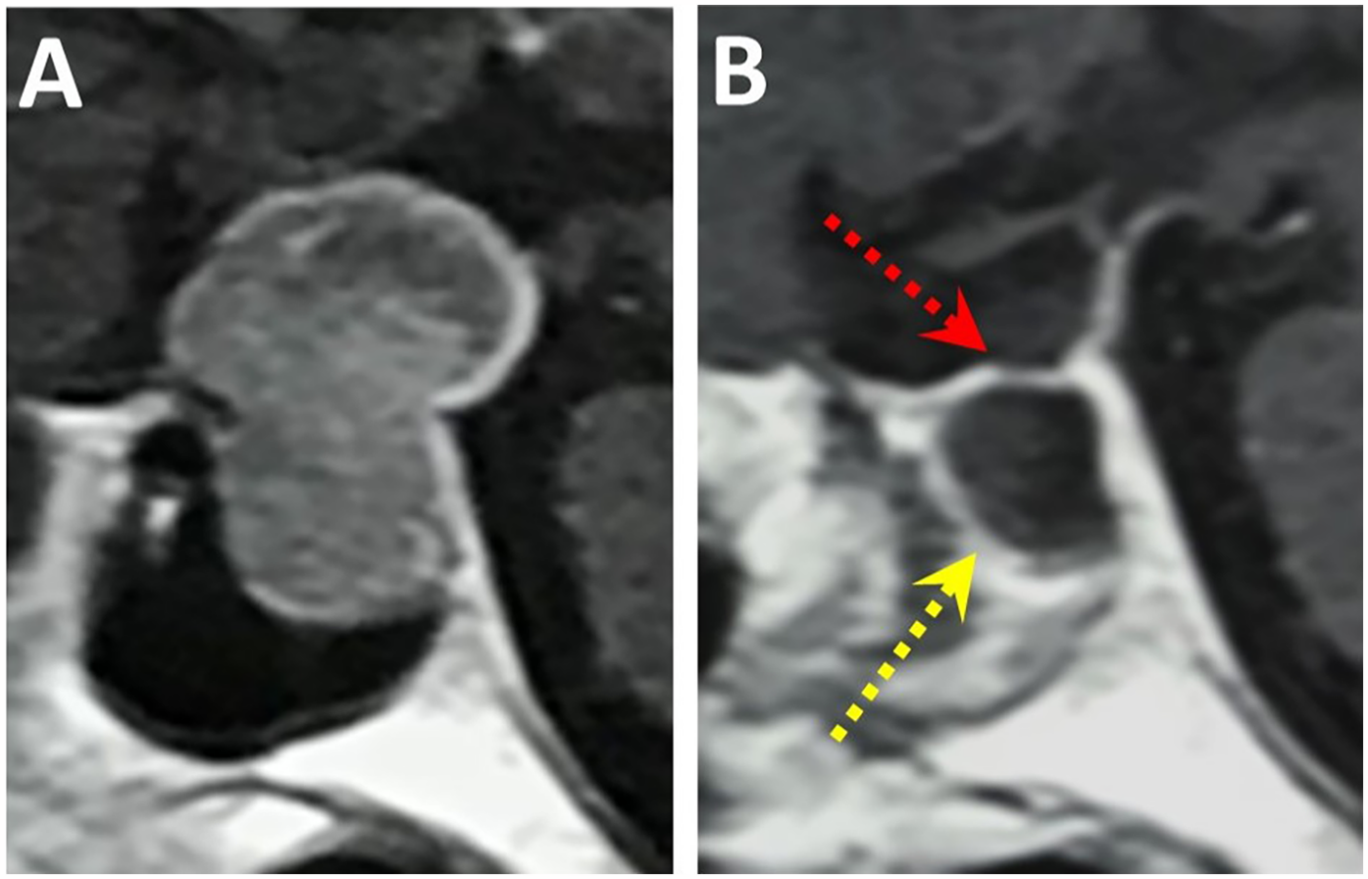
Pituitary tumor MRI before and after surgery (A), preoperative T1-enhanced sagittal view showing pituitary tumor occupancy in the sellae area. (B) Postoperative review at 6 months showed good free mucosal growth (yellow arrow) and intact diaphragma sellae (red arrow).
